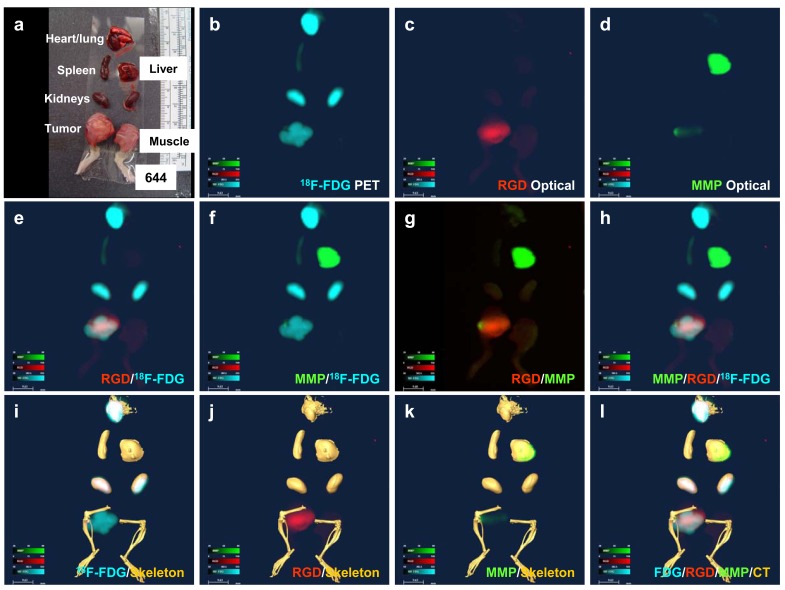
Organ imaging of liver cancer. (a) The color image shows the organ layout. (b) ^18^F-FDG PET imaging demonstrates
